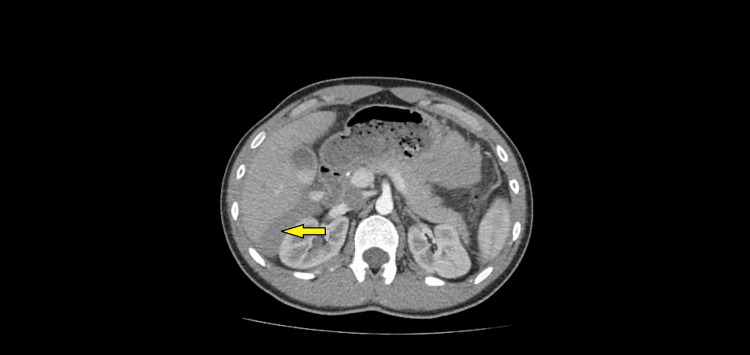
An axial CT image of the abdomen demonstrating high-density fluid, on the right side, in Morrison's pouch (denoted by a yellow arrow)
